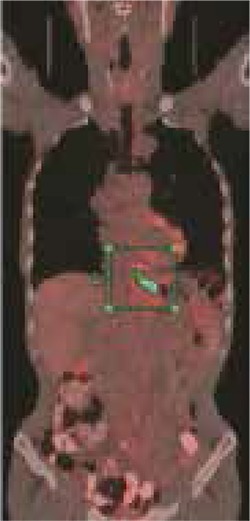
66 year old male patient whose distal esophageal lesion was reported as squamous cell cancer by endoscopic biopsy. 18F-FDG PET/ CT showed FDG uptake in distal esophageal lesion with 14.7 SUV_max_ and MTV was calculated as 23.90 cm^3^.
